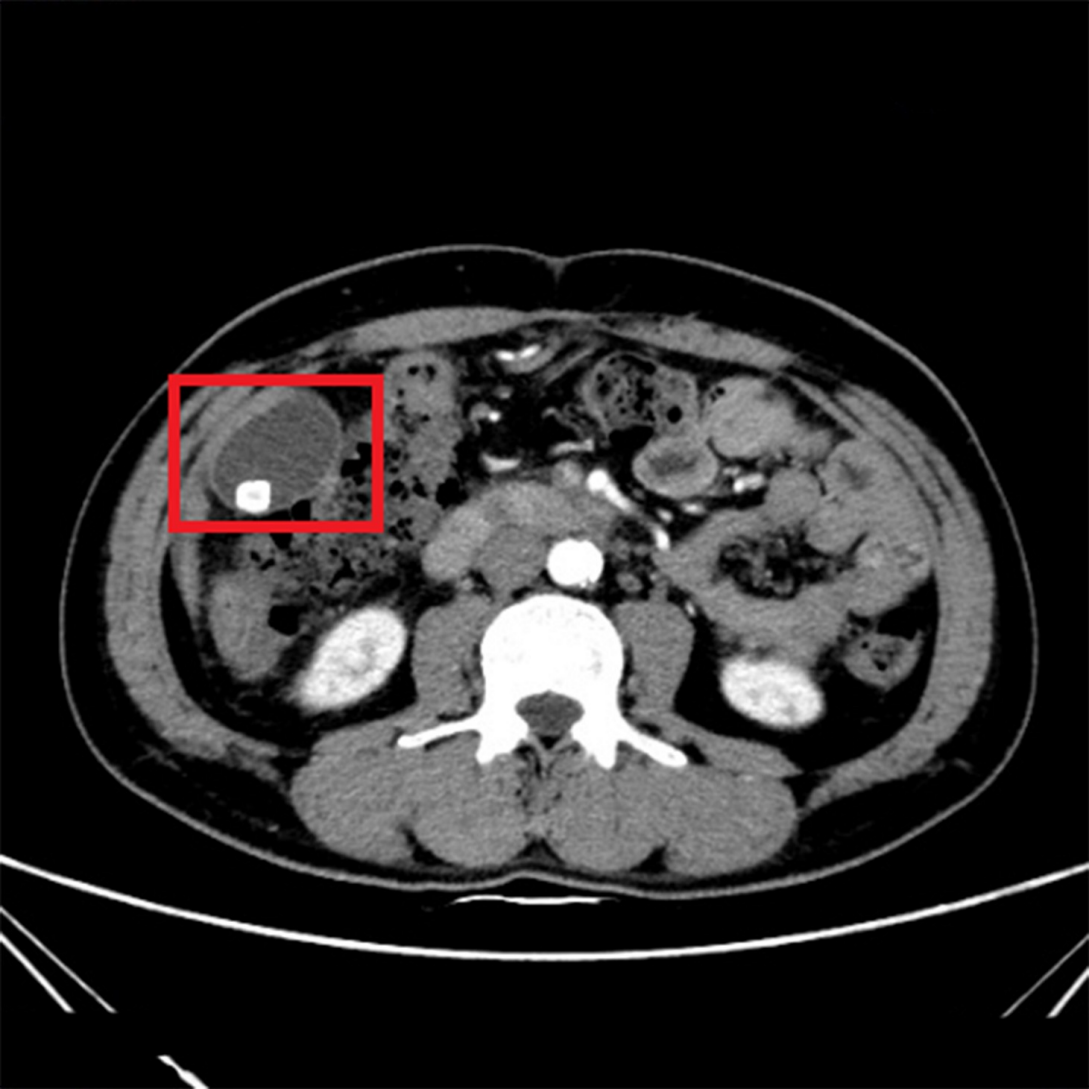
The original CT images of a person with the cholelithiasis.
